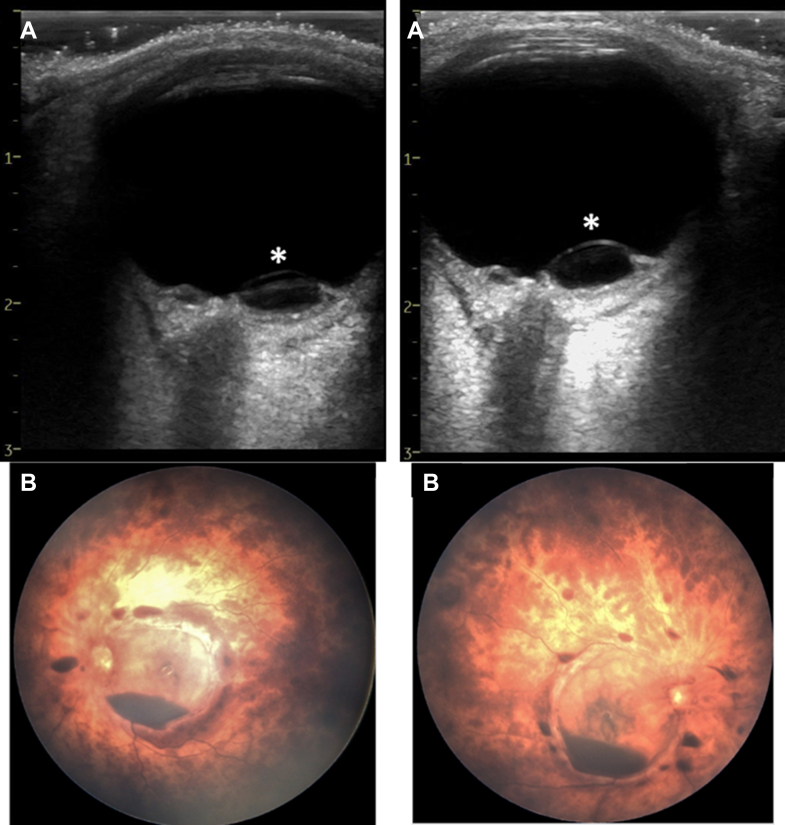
(A) The eye POCUS image with the bulging of the retina posterior wall due to a detachment of the posterior hyaloid called retinoschisis (∗). (B) The corresponding fundoscopic exam confirming the retrohyaloidal hemorrhage with a blood level and retinal fold. POCUS, point-of-care ultrasound.
